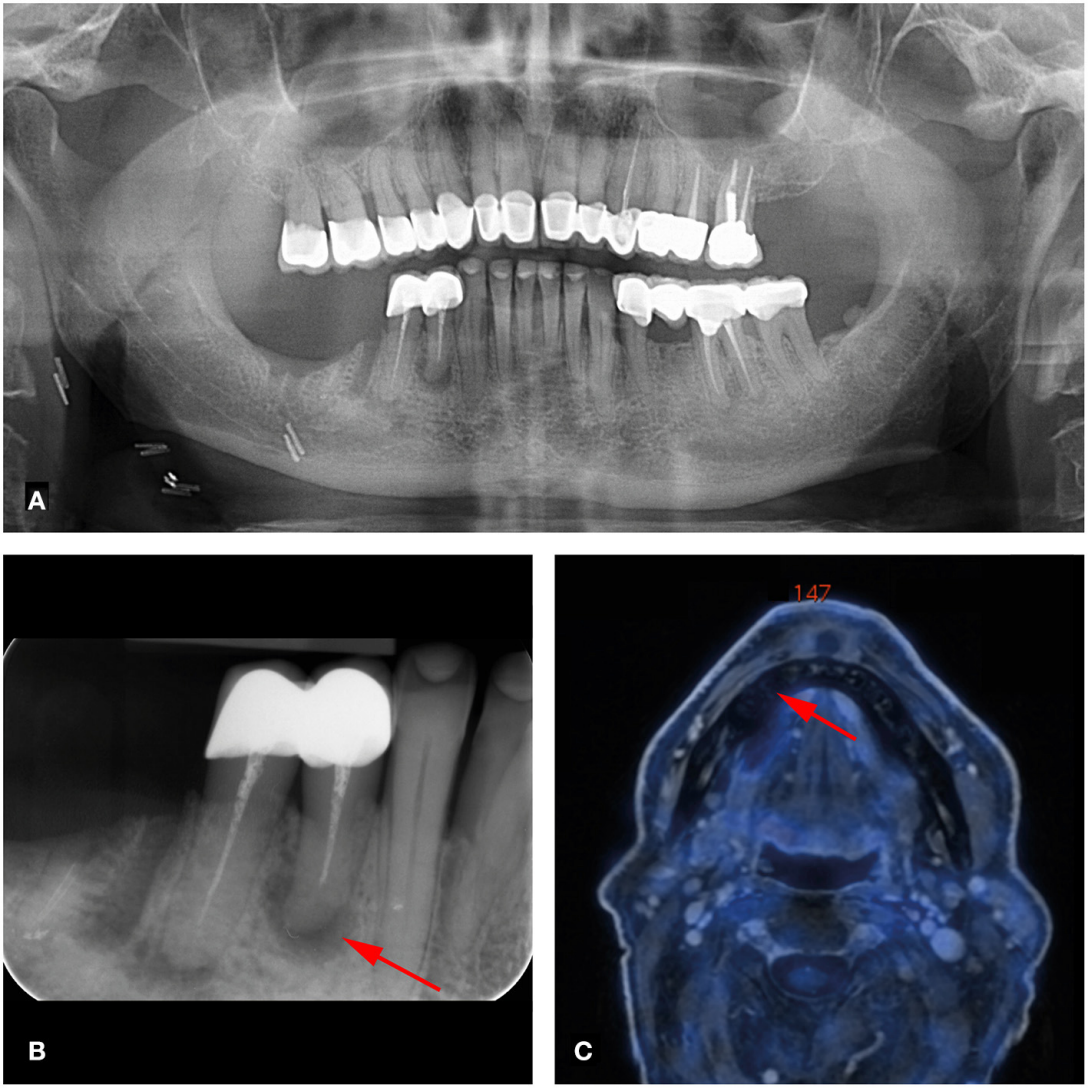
66-year-old man with right-sided oral cavity squamous cell carcinoma cT1 cN2b cMx. Panoramic (A) and dental radiography (B) shows a periapical lesion of tooth 44 of less than 5mm (arrow; PAI score 4, MPI score 1). (C) FDG-PET/MR shows no increased metabolic activity of this lesion (arrow).
